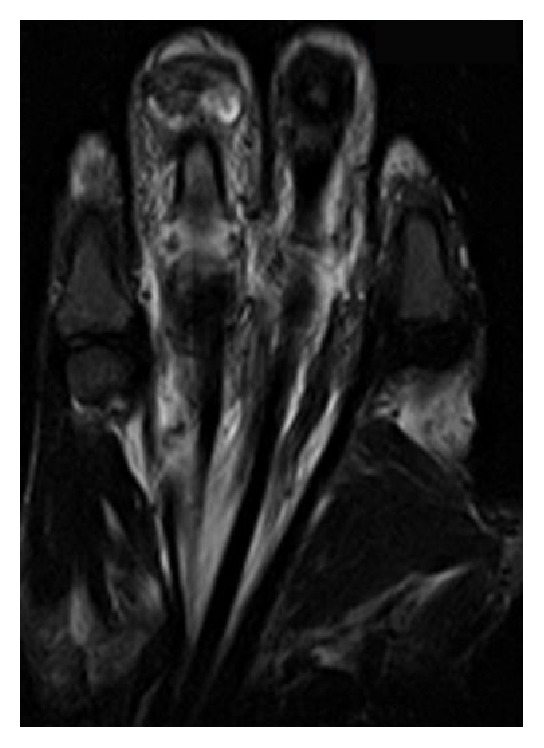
MRI coronal view, edema along tendons.
